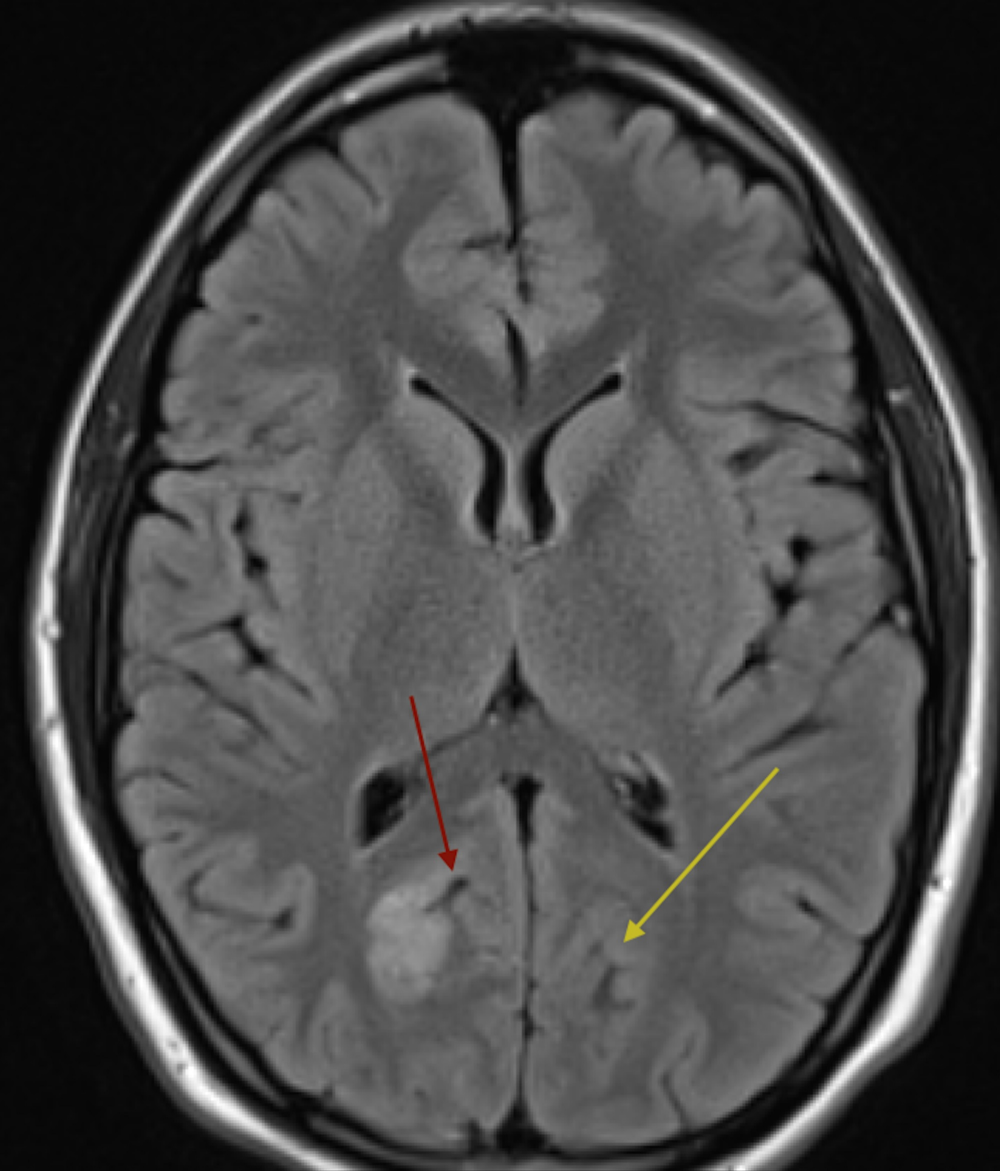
Axial FLAIR MRI demonstrates ischemic infarct obscuring right parieto-occipital sulcus (red arrow) and conspicuous left parieto-occipital sulcus (yellow arrow). FLAIR, fluid-attenuated inversion recovery
